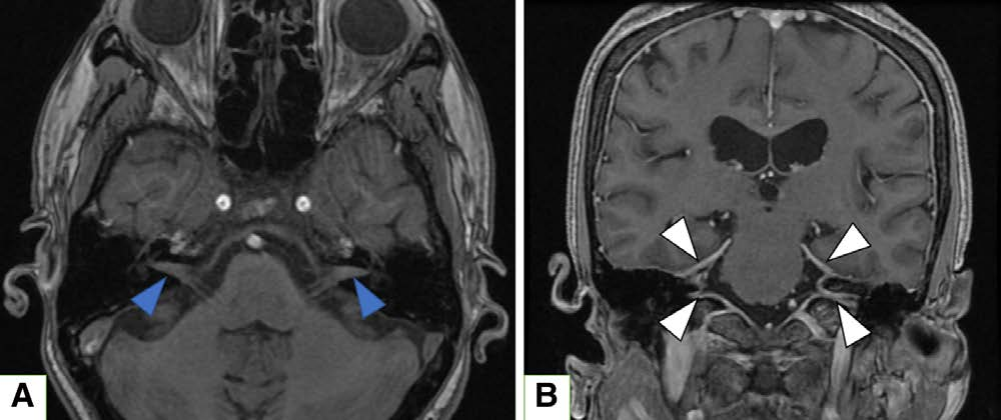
Head contrast-enhanced magnetic resonance imaging (MRI). (A) Occupying lesions in the bilateral internal auditory canals (blue arrows) (Fast Imaging Employing Steady-state Acquisition) (B) Diffuse dural thickening from the middle to the posterior cranial fossa (white arrow) (Contrast-enhanced T1-weighted Images).
